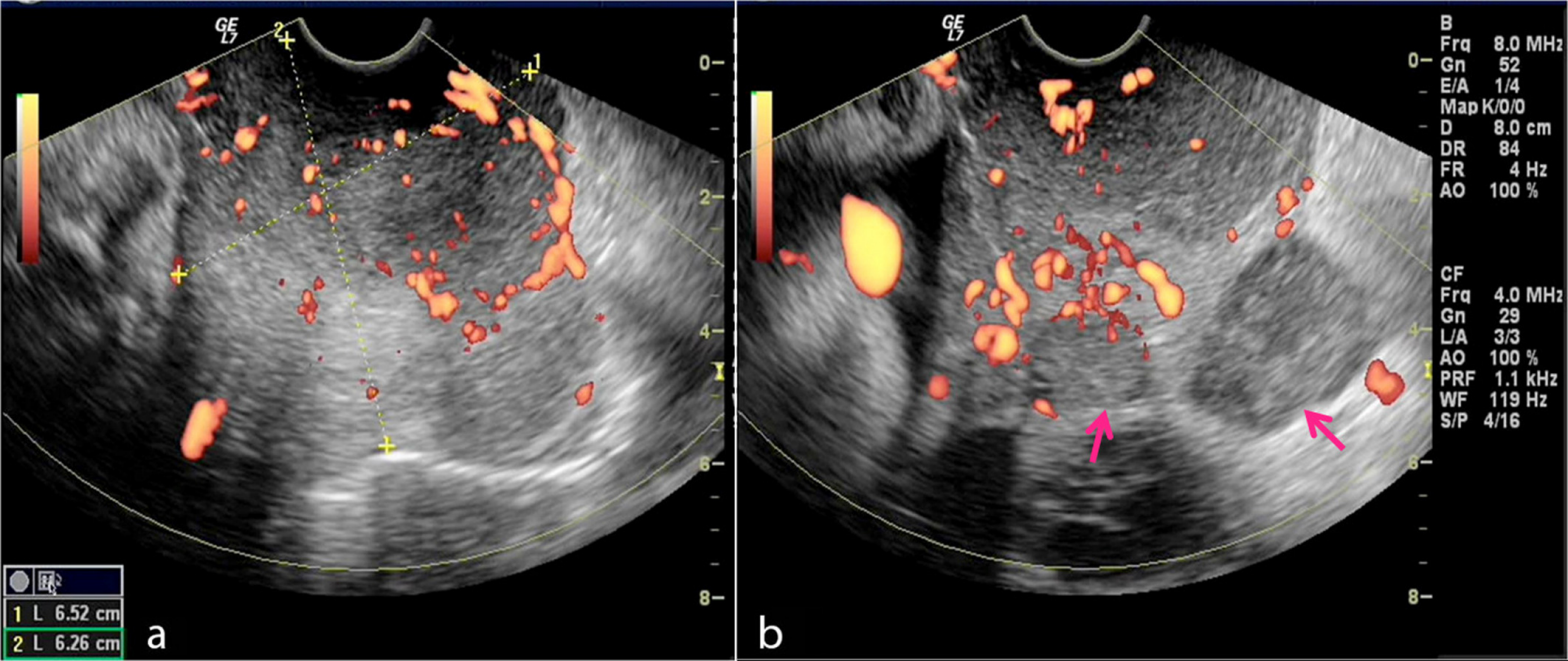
Transvaginal ultrasound with power Doppler imaging shows a bulky highly vascular cervix (a) and polypoidal masses seen in the upper vagina. (b) Peritoneal deposits are seen in the Douglas pouch (arrow).
